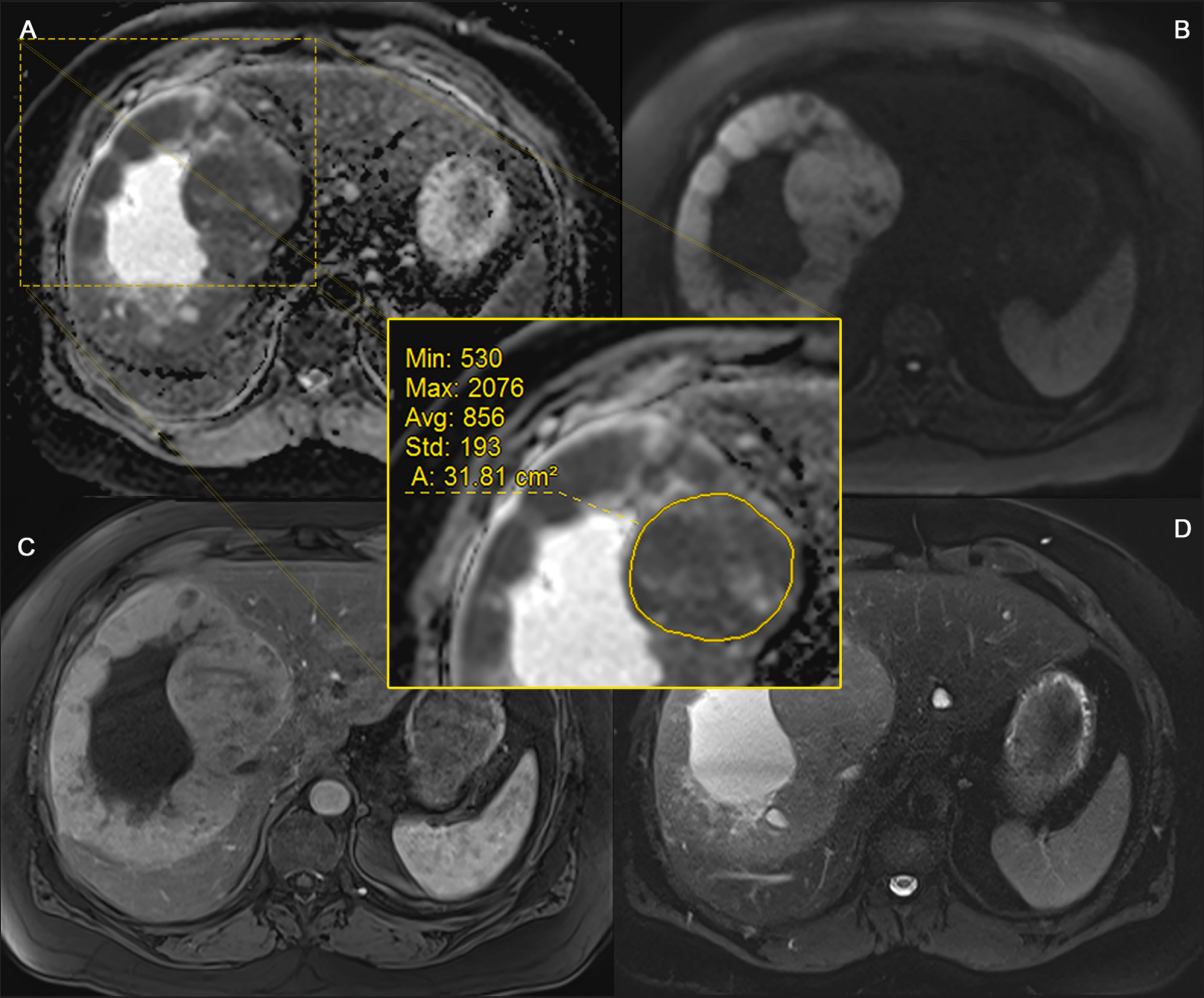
ROI placement (grade 3). Axial ADC map (A), DWI b1000 (B), arterial phase T1-weighted (C) and fat-suppressed T2-weighted (D) images of a biopsy proven G3 NET (primary tumor location = pancreas) in a 55-year-old male. Images show a large liver metastasis with cystic/necrotic centre: ROI placement in the border of the lesion, avoiding the cystic portion and the outermost edges.
